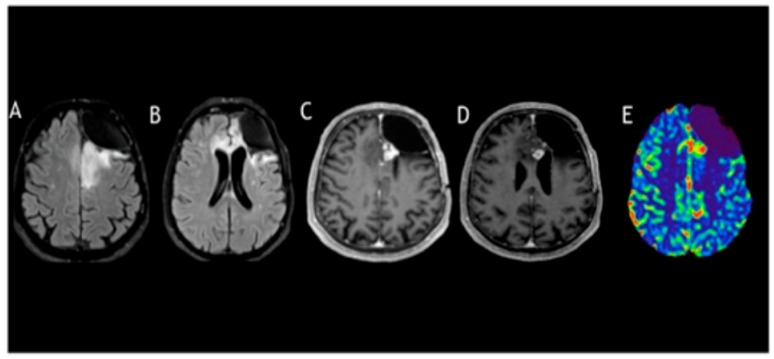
Follow-up MRI at 24 months after surgery and post-radiotherapy. The images shown are MRI FLAIR (A,B), post-contrast T1-weighted images (C,D), and DSC perfusion (E). Tumor progression is evident, with an increase in the infiltrative lesion (A,B) and areas of contrast enhancement (C,D) associated with elevated cerebral blood volume (E).
